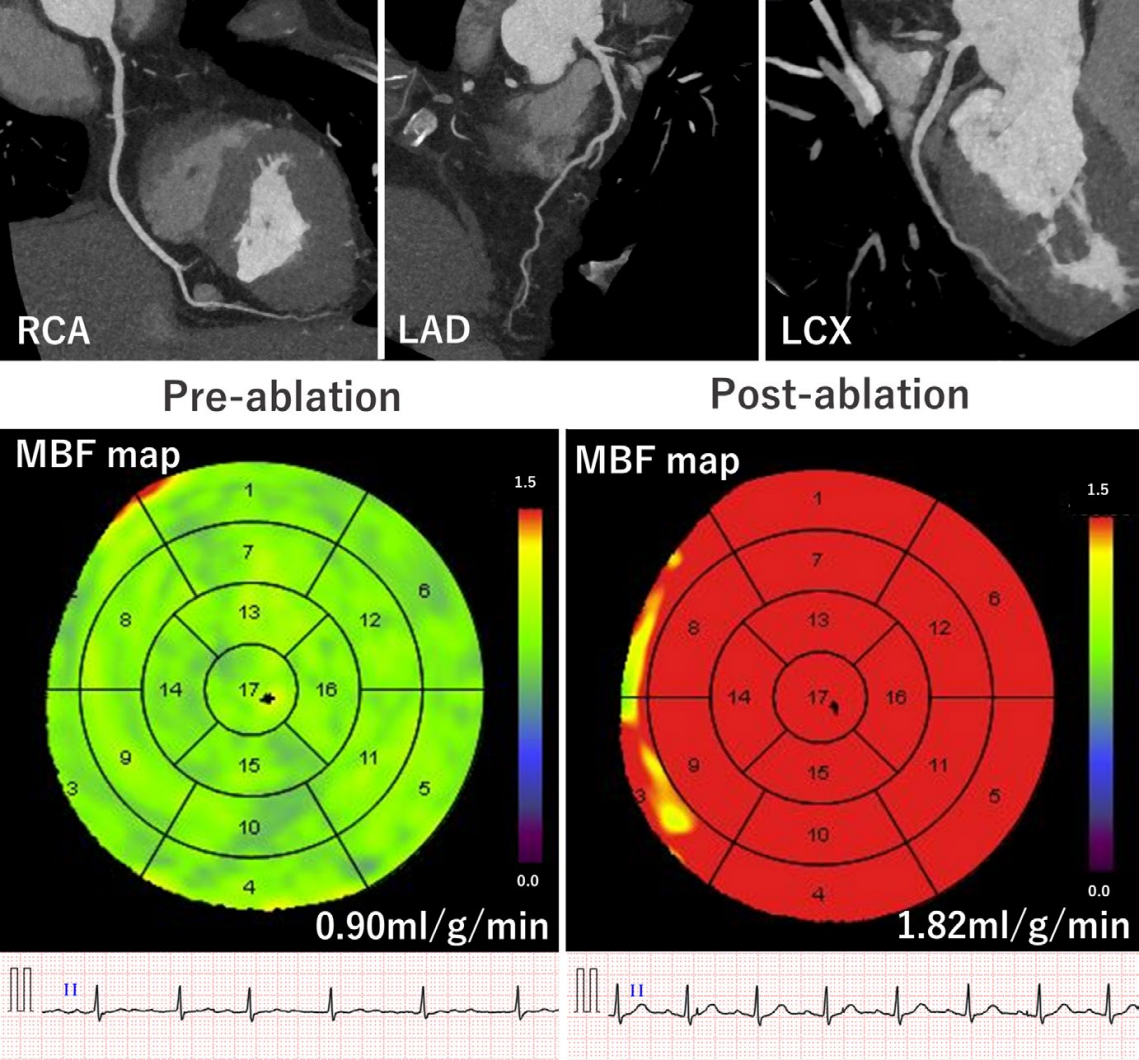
Seventy‐three‐year‐old man with persistent AF who underwent catheter ablation. Heart rhythm was AF during pre‐ablation CT and SR after ablation. Global impairment of hyperemic MBF observed before ablation despite disease‐free coronary arteries (CAD‐RADS score: 0) improved after restoration of sinus rhythm by catheter ablation. Abbreviations are the same as in Figure 3
